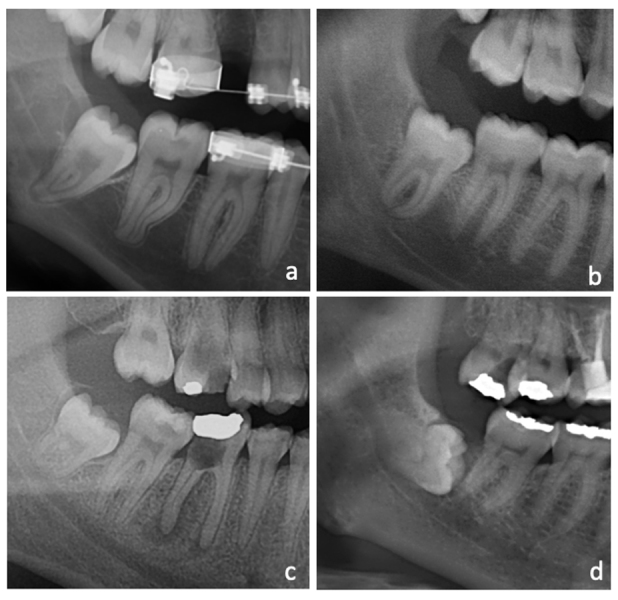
Cutouts of panoramic radiographs showing the absence of marginal bone loss between the second molar and the adjacent third molar (a), slight initial bone loss (b), moderate bone loss reaching the middle third of the second molar root (c) and severe bone loss reaching the apical third of the second molar root (d).
